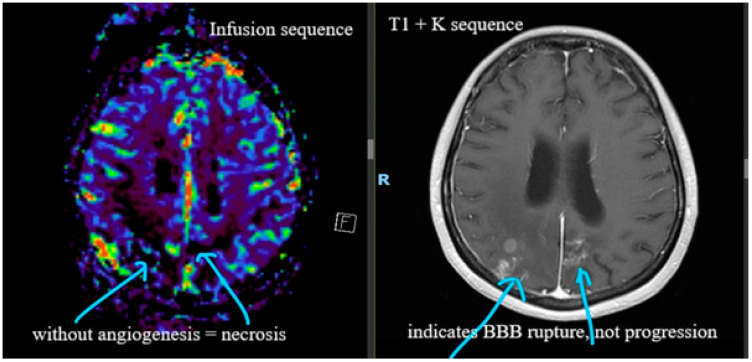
May 2025. Brain perfusion MRI indicating blood–brain barrier (BBB) rupture and no progression.
